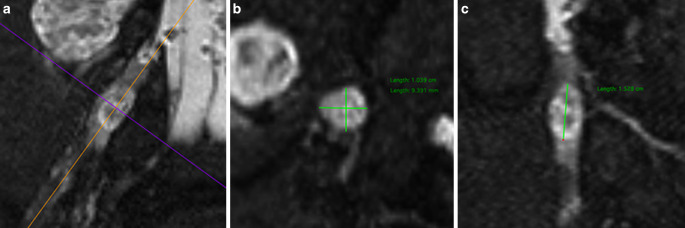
Measurement of DRG volume. DRG volume was assessed in the T2-weighted 3D-image by using the mathematic equation for volume assessment of an ellipsoid: volume in mm^3^ = π/6 * (A × B × C), where A represents the diameter in axial plane (b), B the diameter at 90° in the axial plane (b), and C the length in a reformation along the long axis of the DRG (c), which was determined individually of each DRG (a)
